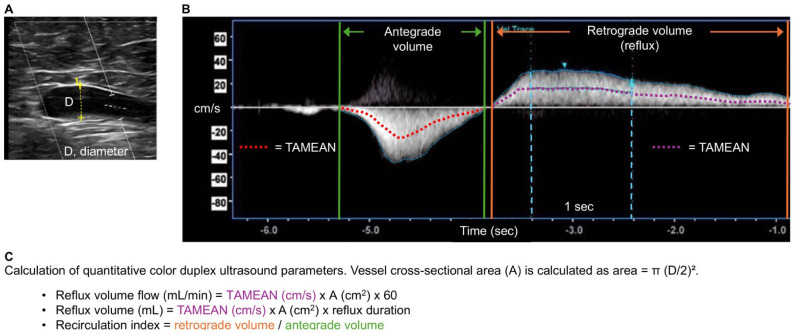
Quantitative assessment of venous reflux using color duplex ultrasound (CDU). (A) B-mode ultrasound image showing measurement of vessel diameter. (B) Example of a pulsed Doppler ultrasound measurement in the proximal great saphenous vein. An automatic compression cuff is inflated to 100 mmHg, resulting in an antegrade flow signal. Upon cuff deflation, a larger retrograde flow (reflux) is observed. Time-averaged mean velocity (TAMEAN) is indicated for both antegrade and retrograde flow phases. (C) Formulas used to calculate quantitative CDU parameters. By measuring the vessel diameter in conjunction with Doppler parameters, reflux volume flow, reflux volume, and the recirculation index can be calculated. Reflux volume flow is calculated using TAMEAN measured over 1 second during the period of highest flow following distal compression release. In contrast, reflux volume is calculated using TAMEAN measured over the entire reflux duration. The recirculation index expresses the ratio of retrograde to antegrade volume.
